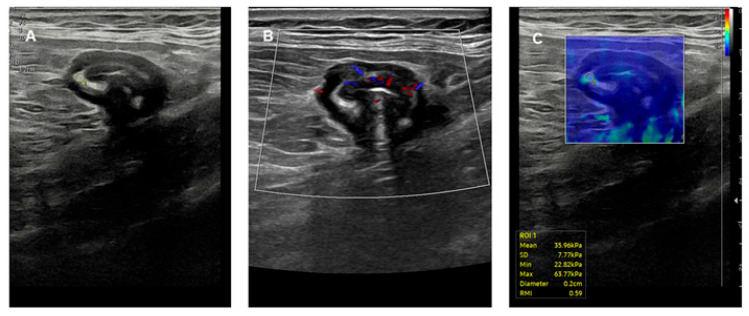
Longstanding left-sided ulcerative colitis under treatment with vedolizumab and in clinical remission in a 70-year-old female patient. Intestinal ultrasound showed a thickened bowel wall of the sigmoid colon with partially preserved stratification at B-mode evaluation (A), mild vascularization at color Doppler (B), and stiff wall at share wave elastography (C), suggesting potential coexistence of fibrosis.
